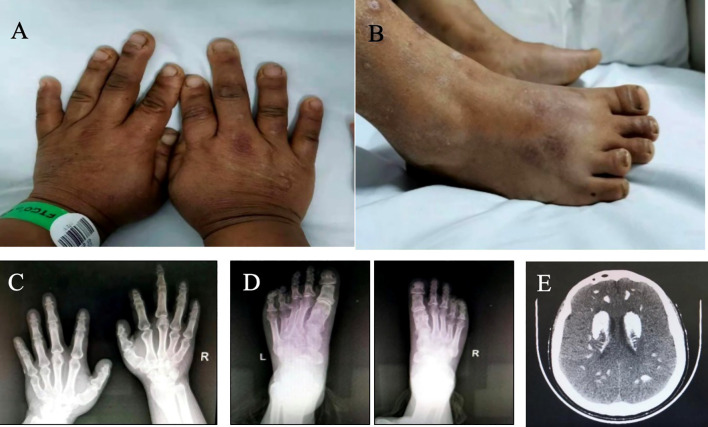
Appearance and imaging of proband 1. (A) hand appearance, (B) foot appearance, (C) X-ray of both hands, (D) X-ray of both feet, (E) CT of the head.
